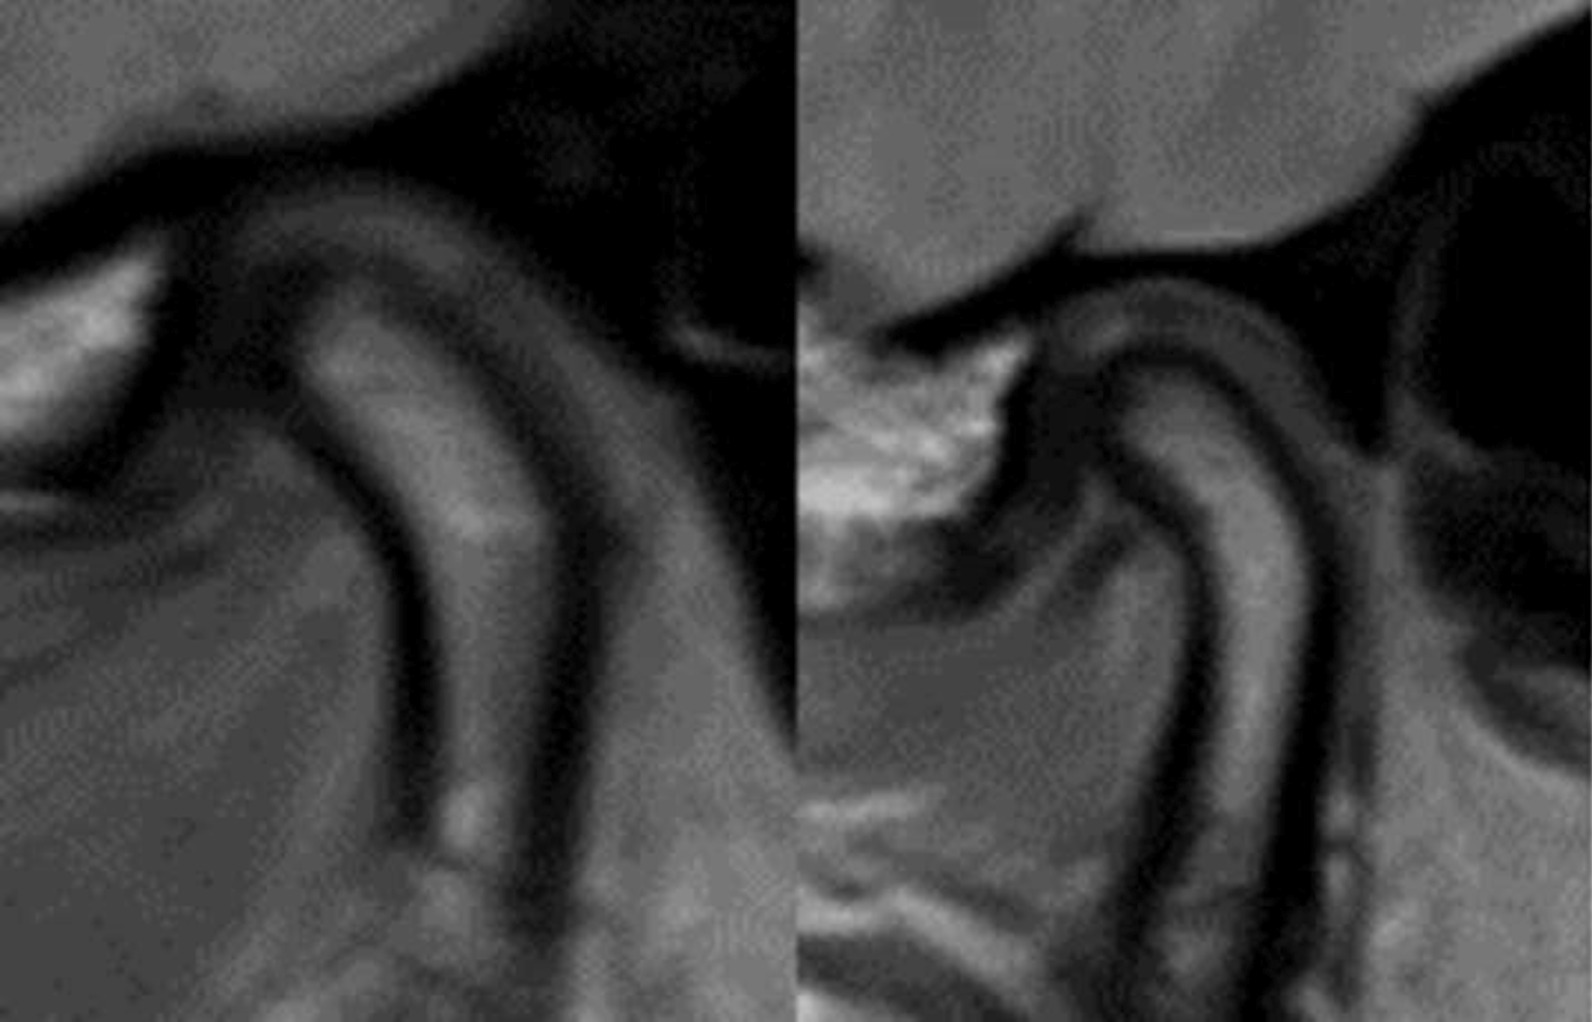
MRI closed mouth left (12/19)
